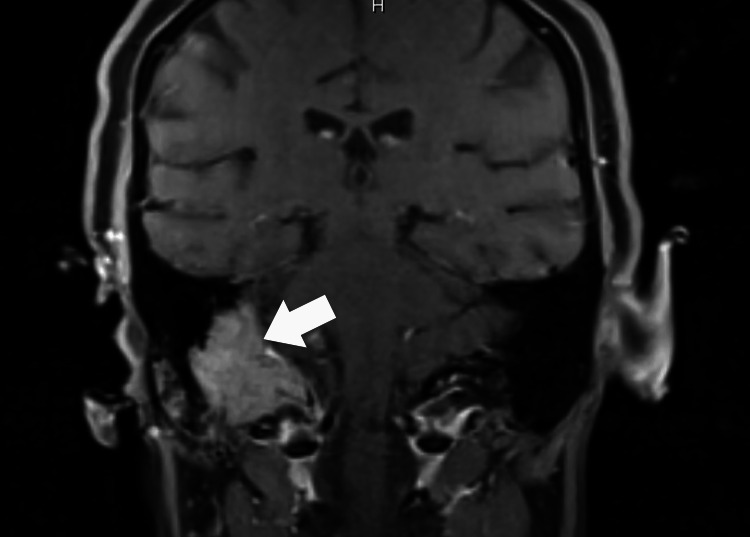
Coronal magnetic resonance imaging with gadolinium contrast of the skull base The arrow points to right skull base with enhancing lesion.
